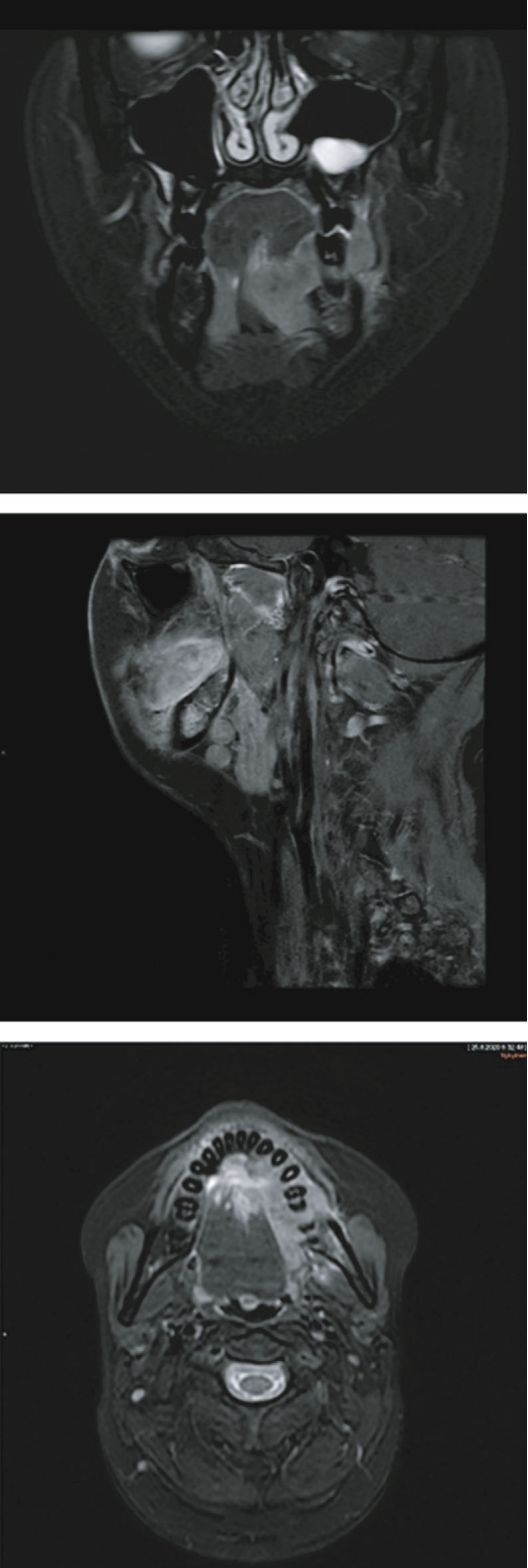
Contrast-enhanced MRI taken after initial examination. The tumor mass is shown infiltrating the mandibular bone, intrinsic musculature of the tongue, and the buccal mucosa, up to the depth of the mylohyoid muscle on the left side. Submandibular pathologic lymph node enlargement is also detected.
